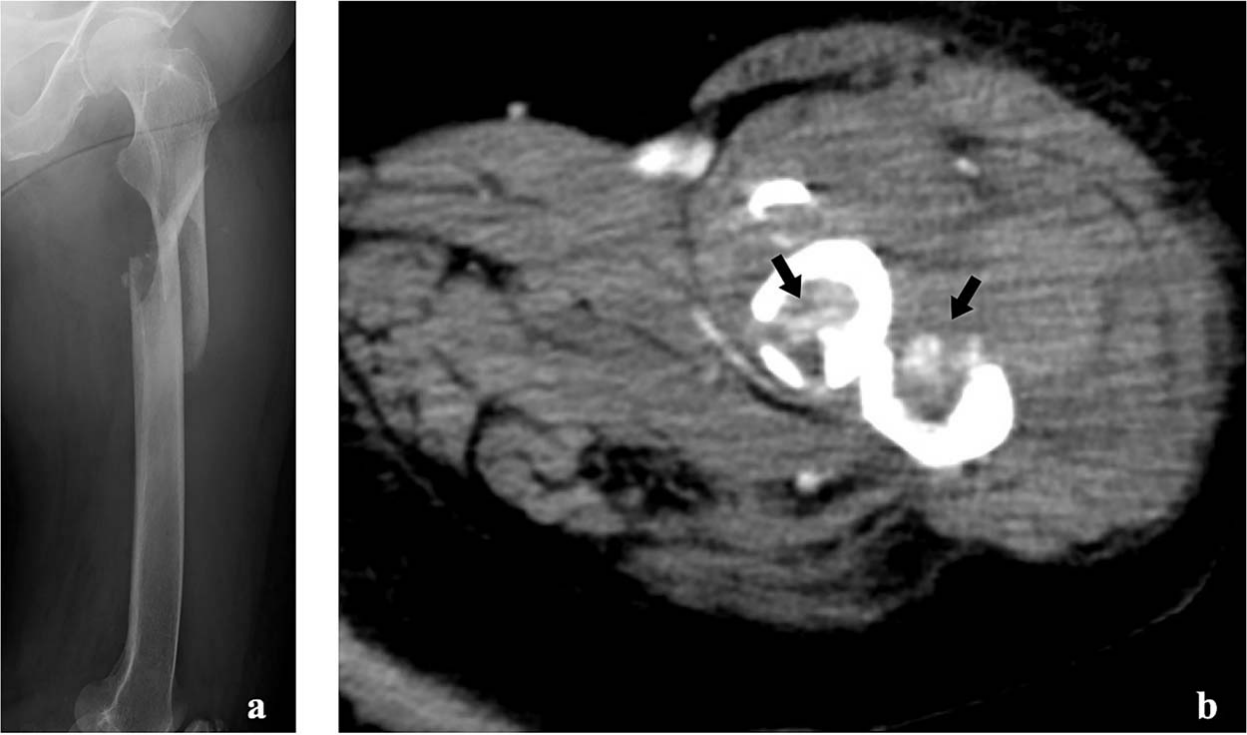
Imaging tests of the left femur. (a) Radiograph showing a fracture of the left femoral shaft. (b) Contrast-enhanced computed tomography showing the tumour strain around the fracture site.
